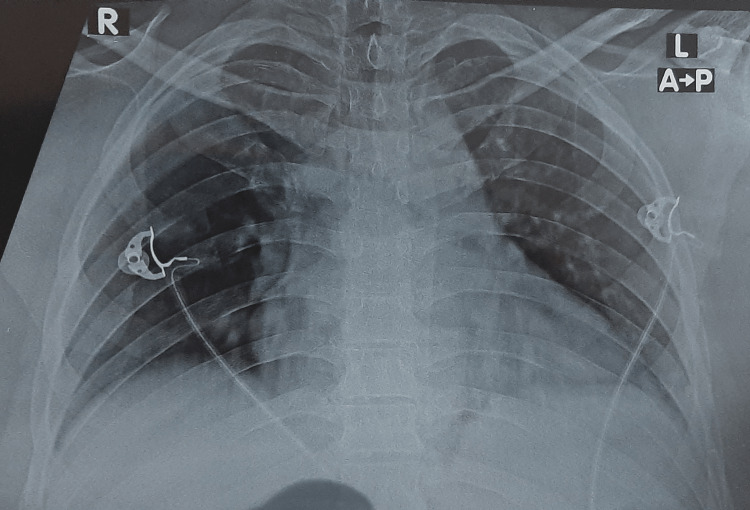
Anteroposterior chest X-ray showing bilateral pulmonary opacification.
